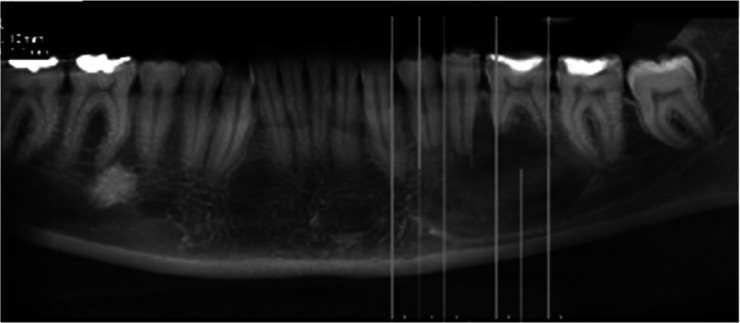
Post-operative cone beam computed tomography (CBCT) image shows normal healing and no signs of recurrence
